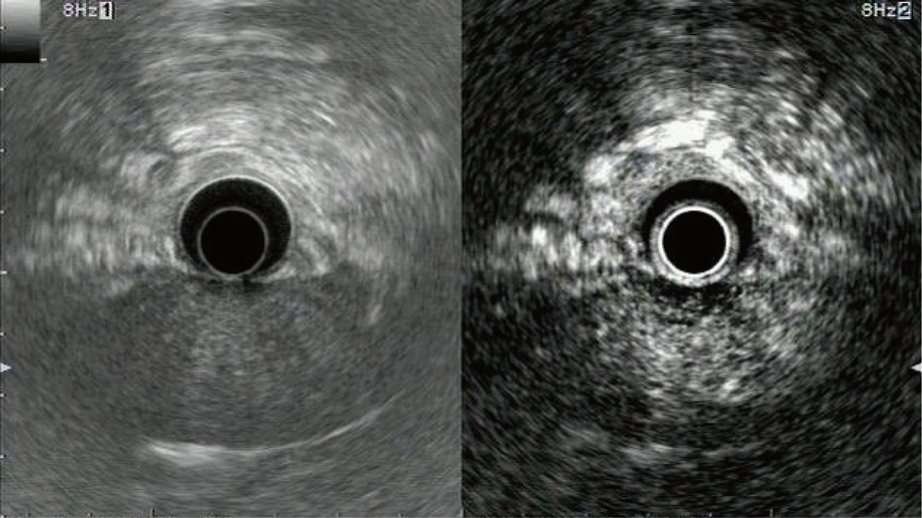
Representative example of focal pancreatitis with hyperenhancement. Conventional endoscopic ultrasonography (left) shows a slightly hypoechoic area without a clear margin at the pancreas head. Contrast-enhanced harmonic endoscopic ultrasonography (right) indicates that enhancement in this area is higher than in the surrounding tissue, and a margin is clearly visible.
